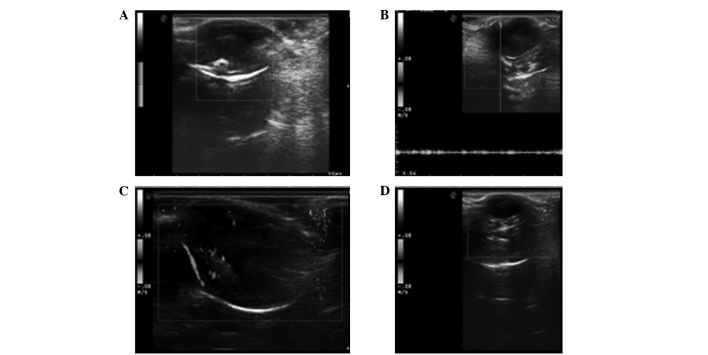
Doppler images showing blood flow changes of the tumours from the (A) NC, (B) ES, (C) RT and (D) ES + RT groups. NC, control; ES, Endostar; RT, radiotherapy; ES + RT, Endostar combined with radiotherapy.
